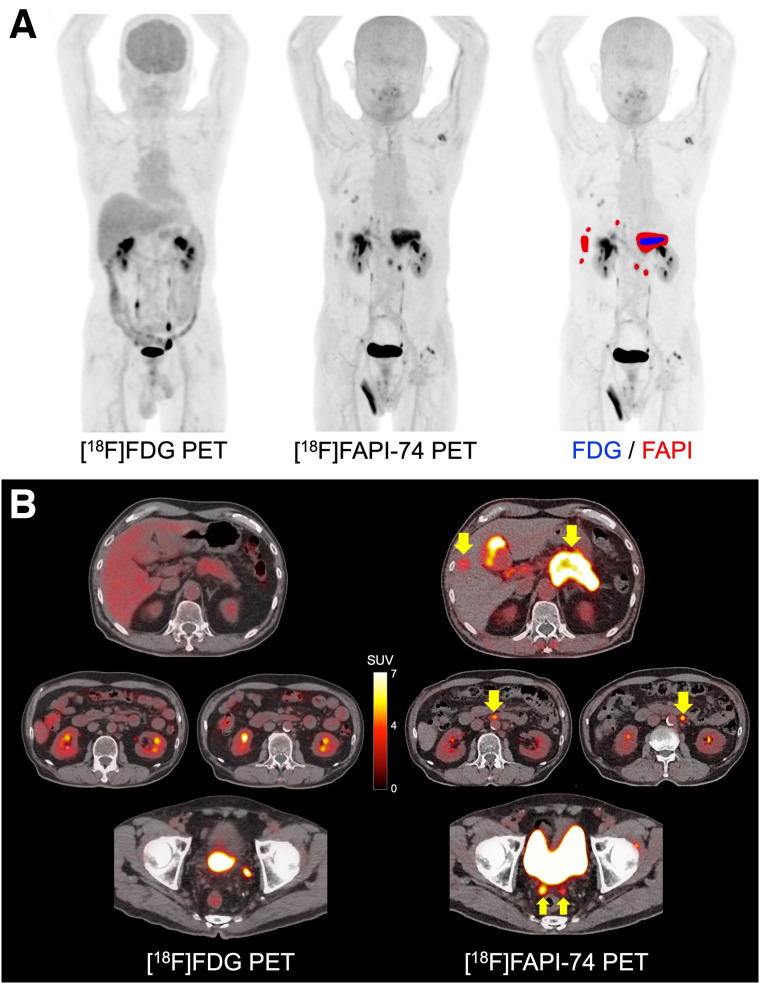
A 68-y-old man with multiple LN and peritoneal metastases of pancreatic cancer. (A) Comparison of maximum-intensity projection images: image on right shows fusion with positive lesions on [^18^F]FAPI-74 PET (red area) and [^18^F]FDG PET (blue area). (B) PET/CT images on [^18^F]FAPI-74 PET and [^18^F]FDG PET (arrows indicate metastatic lesions). [^18^F]FAPI-74 PET detected more metastatic lesions than [^18^F]FDG PET (SUV_max_ of primary lesion is 9.4 and 3.2, respectively). Brain uptake is decreased on [^18^F]FDG PET because of high blood glucose level (277 mg/dL).
